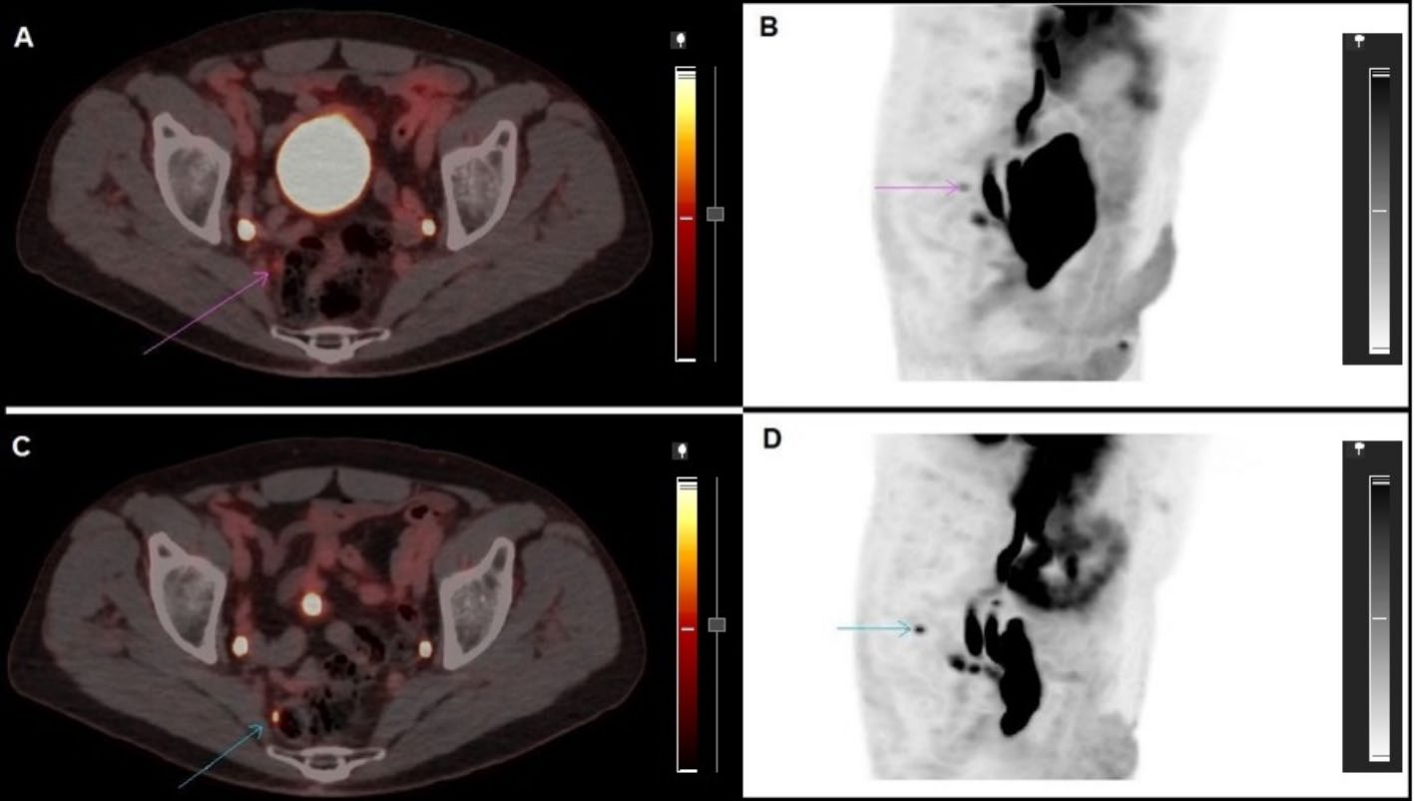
A and B) Axial views and MIP of the early phase PET-PSMA showing a small right pararectal lymph node with mild PSMA expression of uncertain etiology. C and D) Second-stage axial and MIP images showing a moderate increase in PSMA expression in this lymph node and suggesting malignancy. In this patient, the PSA level decreased after RT administration in the referred lymphatic regions
